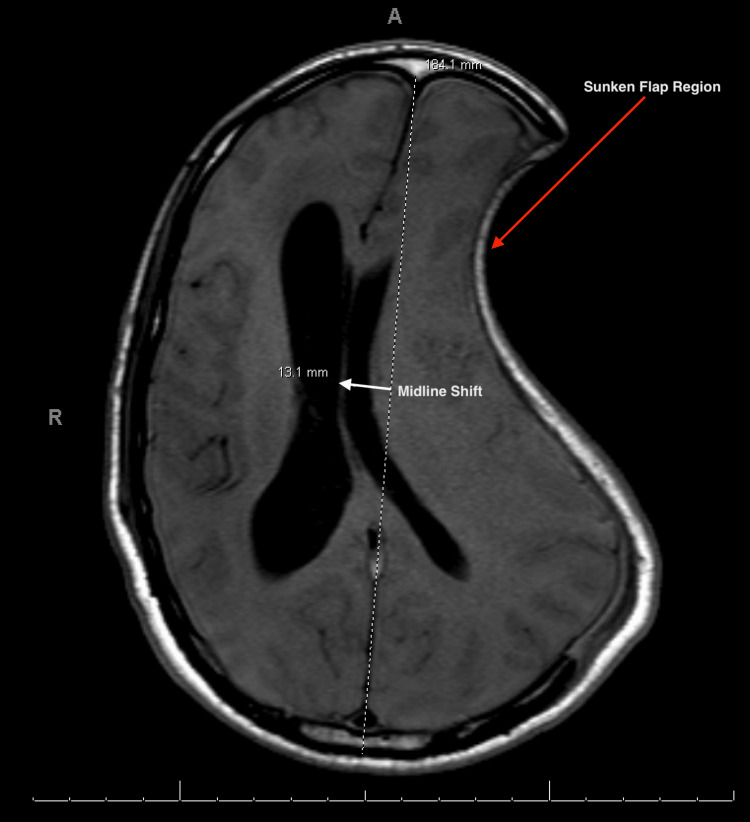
Axial magnetic resonance imaging (MRI) of the brain demonstrating paradoxical brain herniation with approximately 1.3 cm rightward midline shift (white arrow) following decompressive hemicraniectomy, consistent with sinking skin flap syndrome. The sunken flap region is visible along the craniectomy site (red arrow).
